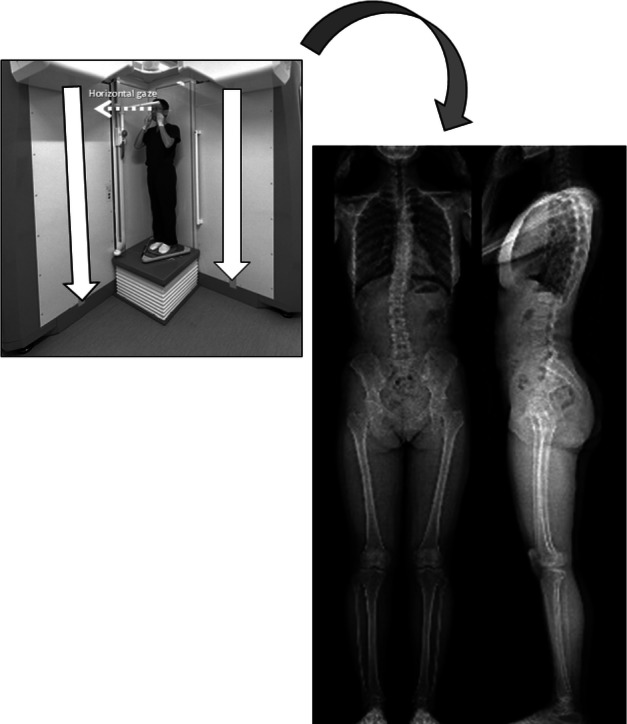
EOS system (a slot-scanning three-dimensional X-ray imager). From the simultaneous anteroposterior and lateral X-rays of the whole body to the 3D bone external envelope technique, 3D reconstruction is possible at every level of the osteoarticular system and especially the spine in the standing position. The subject is the author (K.H.).
